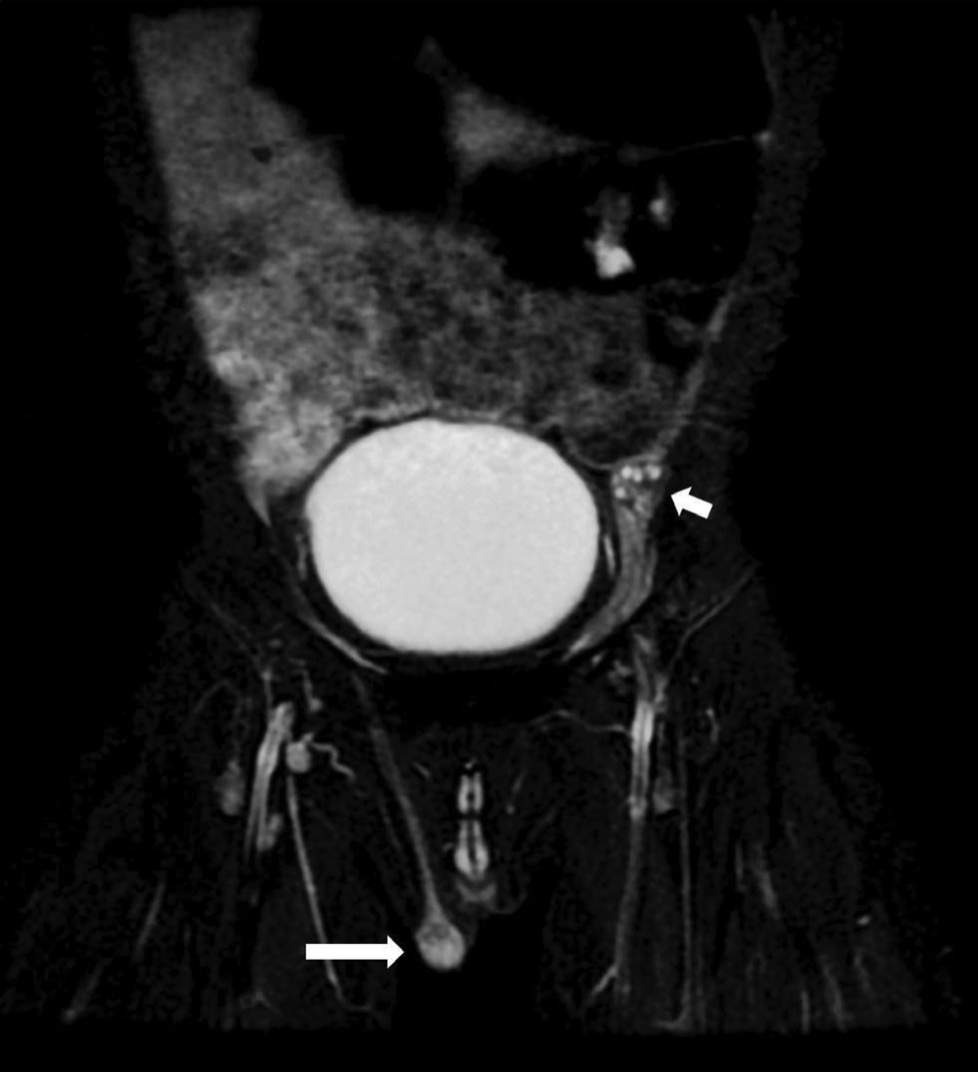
Coronal fat-suppressed T2-weighted image of the magnetic resonance sequence shows testicular tissue in the right scrotum. There is no testicular tissue in the scrotal area on the left. Ovarian tissue with millimetric follicular cysts is present in the left inguinal region
